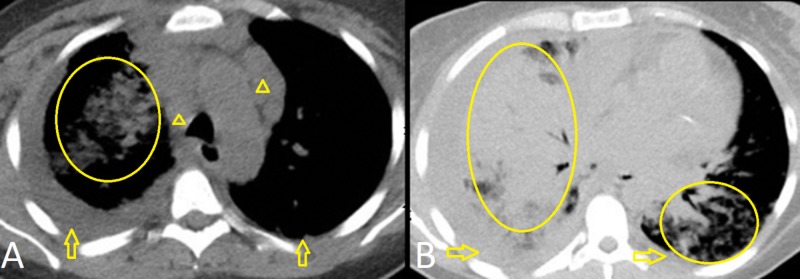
Chest CT of upper lungs (A) and lower lungs (B) showing multifocal bronchopneumonia (oval), moderate right and small left partially loculated pleural effusions (arrow), and enlarged mediastinal and bilateral hilar lymph nodes (arrowhead). Bilateral axillary and supraclavicular lymph nodes were also enlarged.
